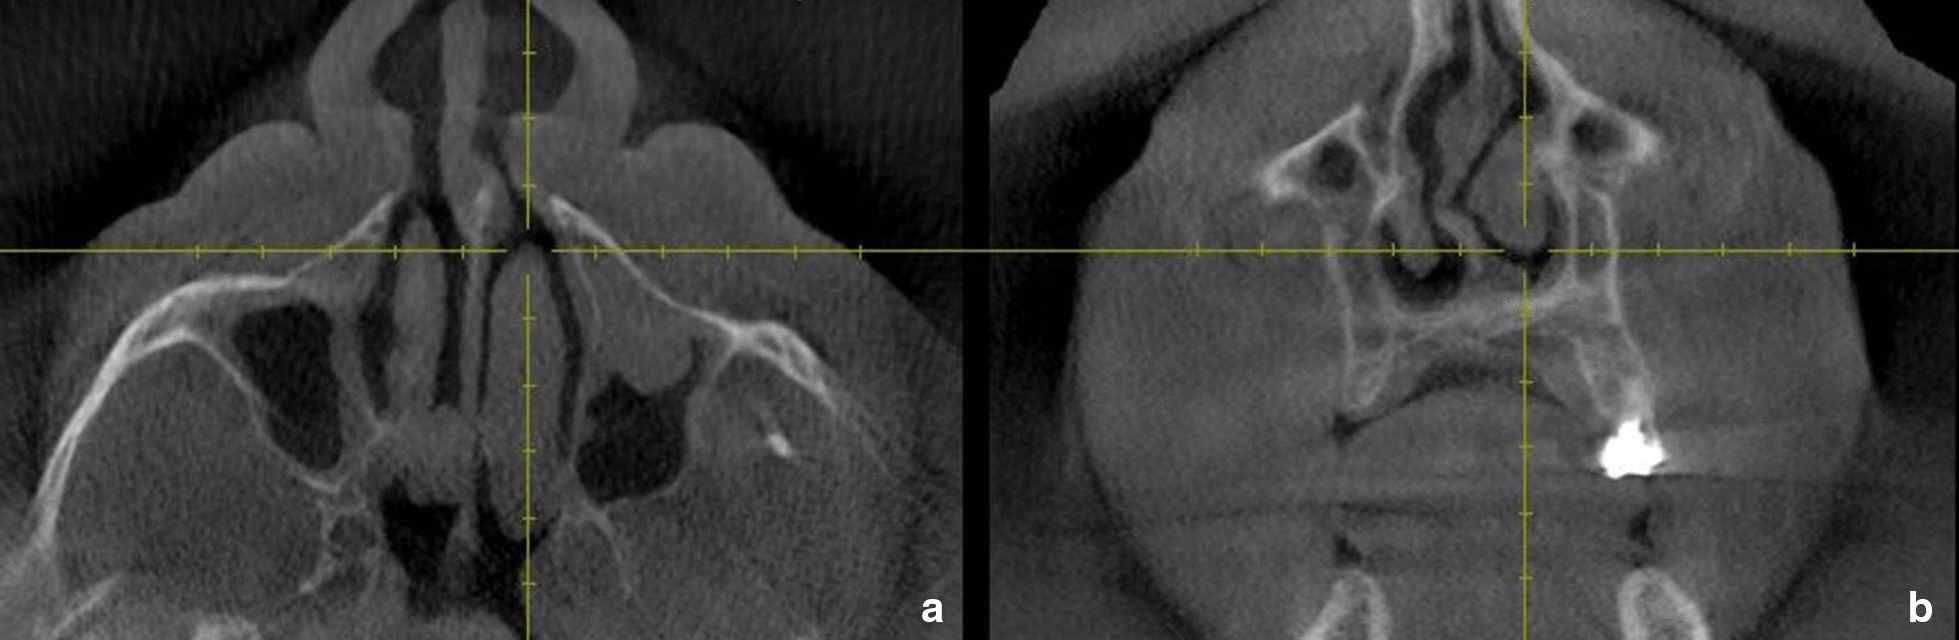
Axial (a) and Coronal (b) CBCT sections showing the site at which the anterior width of the inferior turbinate was determined in the study
